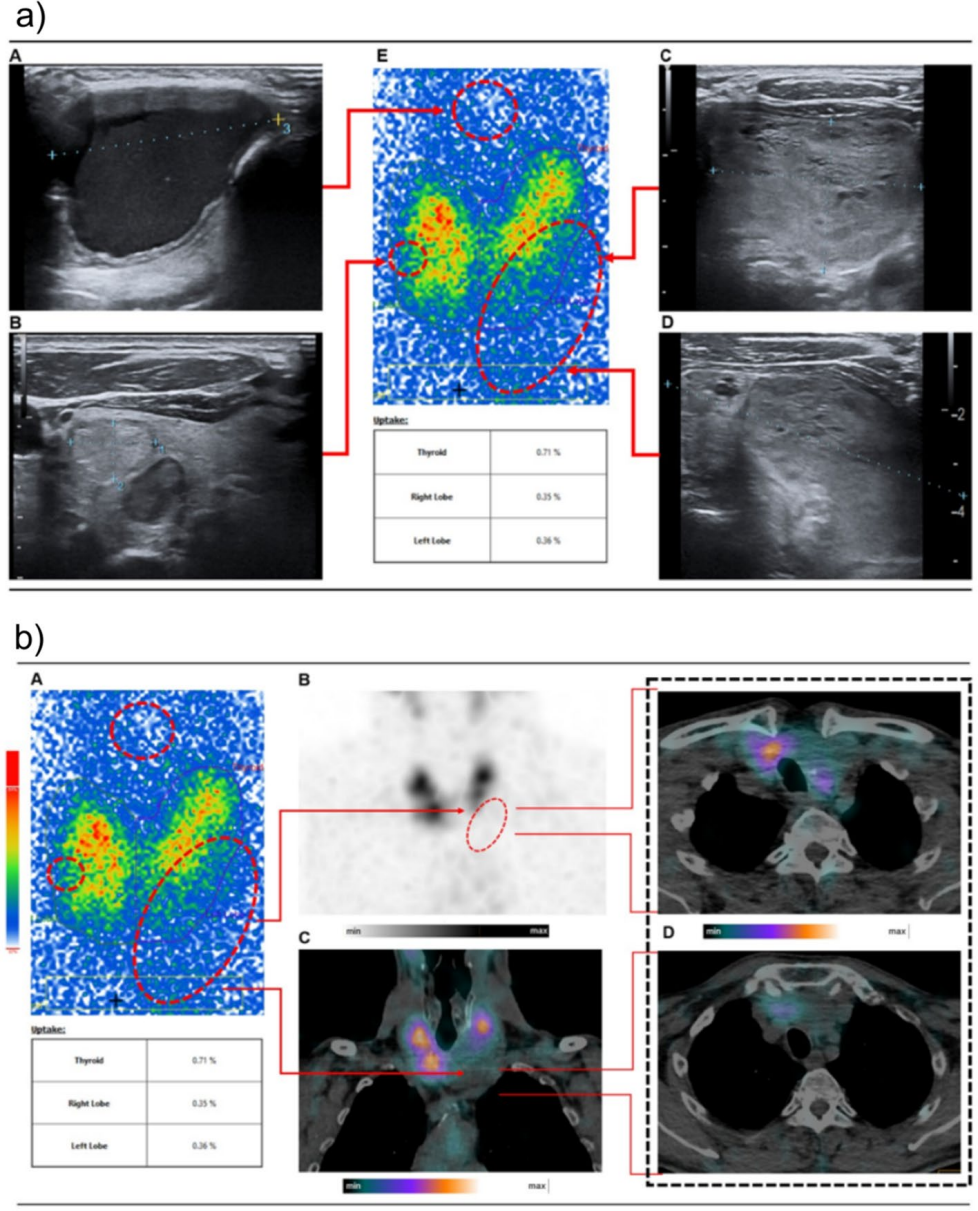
The ultrasound examination revealed solid, clearly margined TN left lower pole of thyroid gland (B, C, D) on a euthyroid goiter. The planar thyroid scan finding was consistent with a hypofunctioning TN (E). Furthermore, the ultrasound examination found a cystical non-thyroid structure incidentally. Figure 3B depicts the thyroid enlargement into thoracic cavity resulting in tracheal stenosis and shift to contralateral side in a 59-year-old-male-patient (A–D) revealed by hybrid imaging
